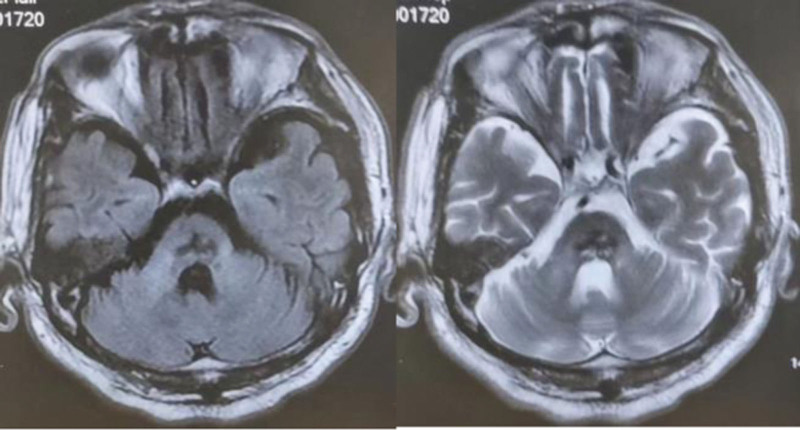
MRI image of brain stem hemorrhage at 5 months, bilateral central tegmental tract is more likely to be damaged. MRI = magnetic resonance imaging.
